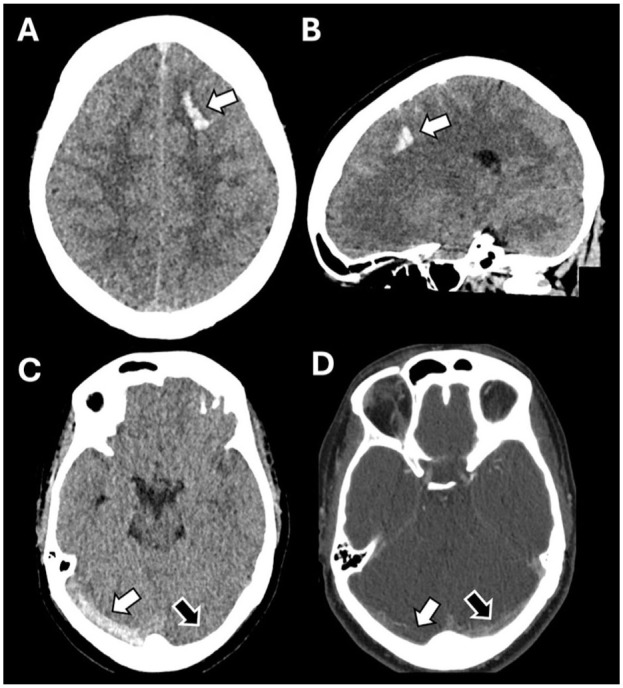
Non-contrast CT images of CVT patients with markers that could help to identify CVT using artificial intelligence. (a/b) A solitary juxtacortical hemorrhage is situated in the frontal lobe of the left hemisphere, confined to the white matter just below the cortex. (c) Patient with a right transverse sinus thrombosis and corresponding dense vessel sign (white arrow). The left transverse sinus demonstrates normal density (black arrow). (d) Contrast-enhanced CT of patient C, with the white arrow indicating the thrombosed sinus and the black arrow demonstrating preserved vessel patency.
